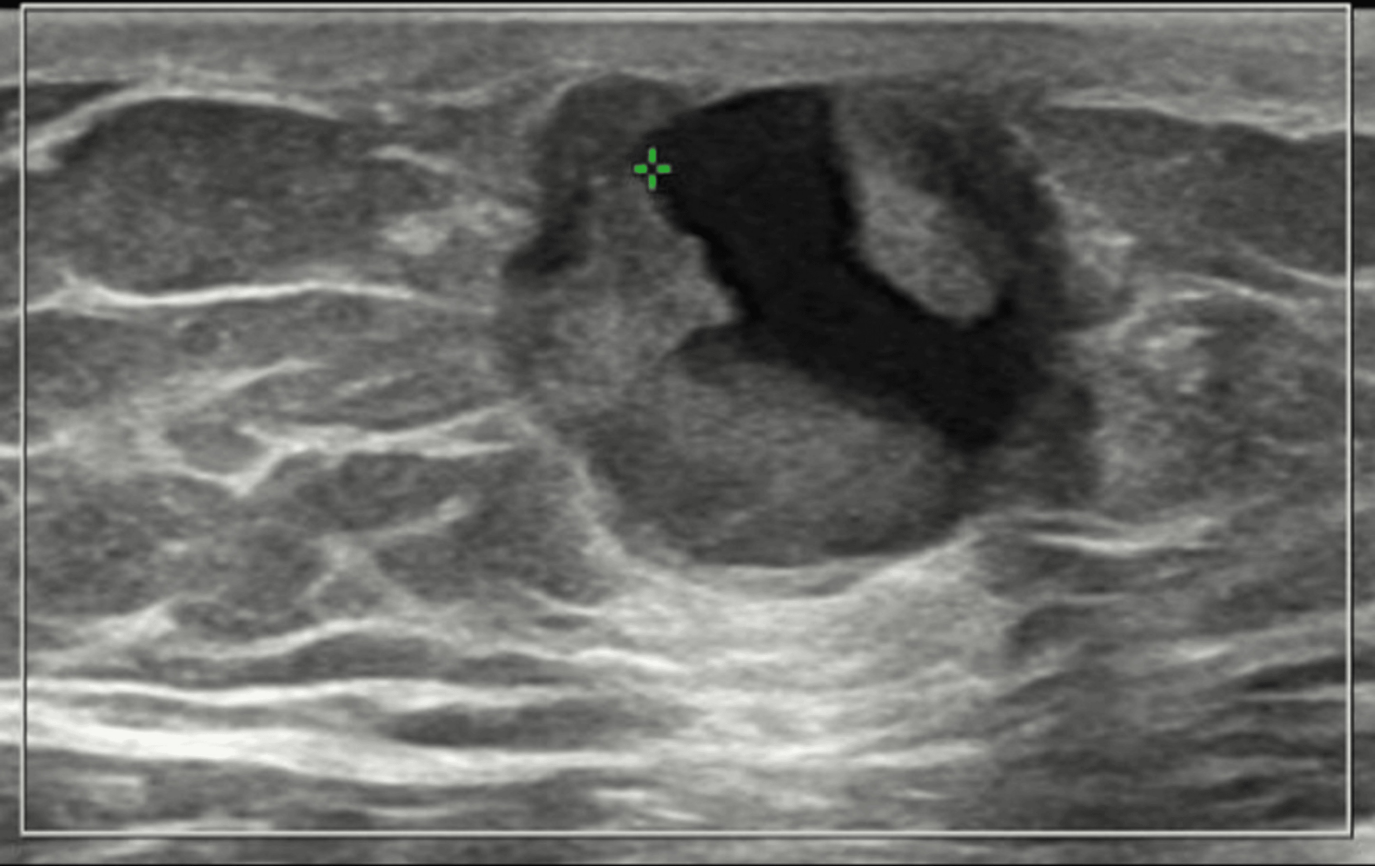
Right breast ultrasonography showing a solid-cystic mass in the right retroareolar region The lobulated solid-cystic hypoechoic mass measured 15 x 27 x 25 mm, located in the right retroareolar area. Doppler imaging revealed vascularity within the mass. Imaging features were consistent with a primary malignant breast lesion. No other lesions in the right breast were seen. Suspicious-appearing lymph nodes were observed in the right axilla.
